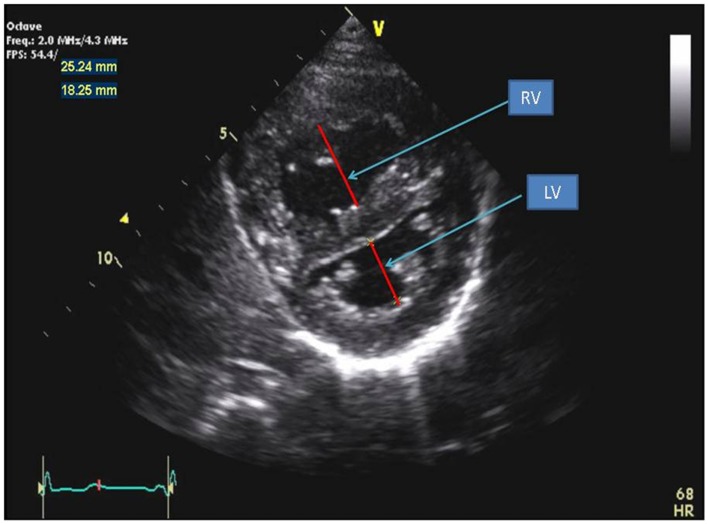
Parasternal short axis view of the right and left ventricles at the level of the papillary muscles. The RV/LV ratio is derived from RV diameter and LV diameter at end-systole.
